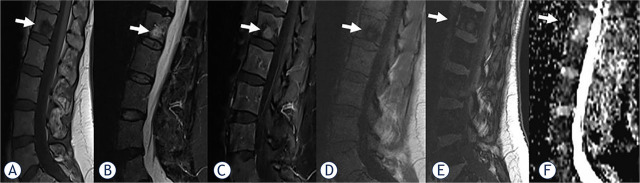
A 50-year-old woman with breast cancer and focal benign vertebral bone marrow lesion (VBML) (arrows). A round-shaped, abnormal signal intensity change in the bone marrow is evident in the L1 body on sagittal T1-weighted (A), short tau inversion recovery (STIR), (B), contrast-enhanced fat- satureted T1-weighted (C) in-phase T1-weighted (D), out-of-phase T1-weighted (E) images. Apparent diffusion coefficient (ADC) (F) value is 1.74 x 10^−3^ mm^2^/s. The signal intensity ratio (SIR) value is calculated as 0.8 and is consistent with benignity. Standardized Uptake Value (SUV) on PET scan excluded malignancy. After one year of follow-up, the lesion STIR/postcontrast hyperintensity almost disappeared.
